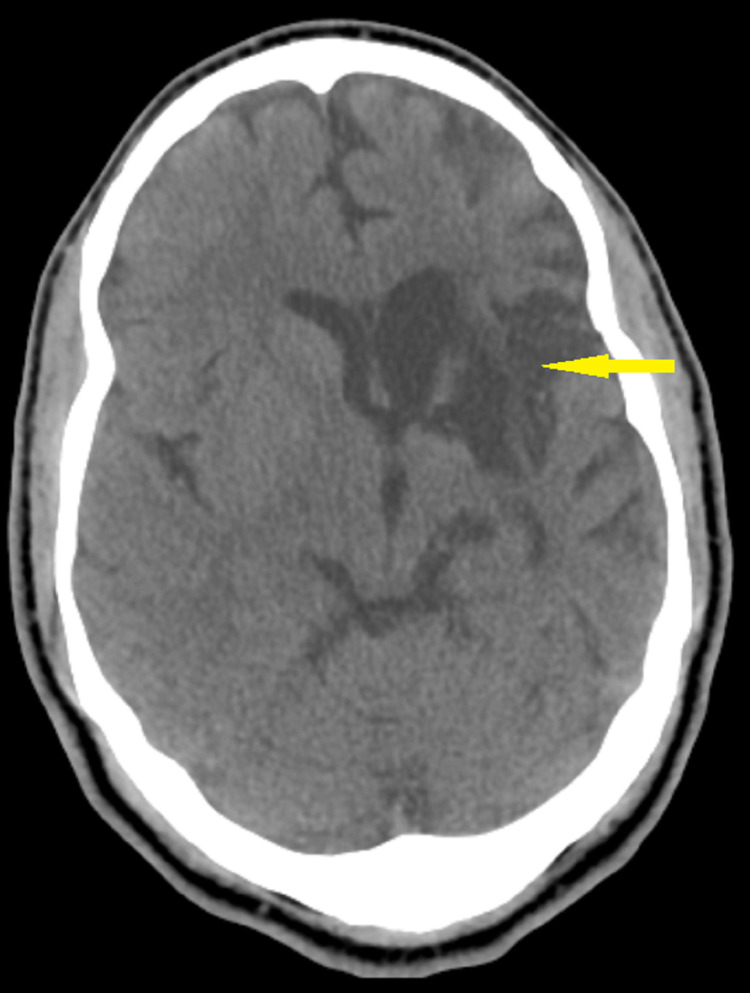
CT of the head showing chronic infarction of the left basal ganglia/corona radiata and the insula and frontal opercular region (6/2021)
